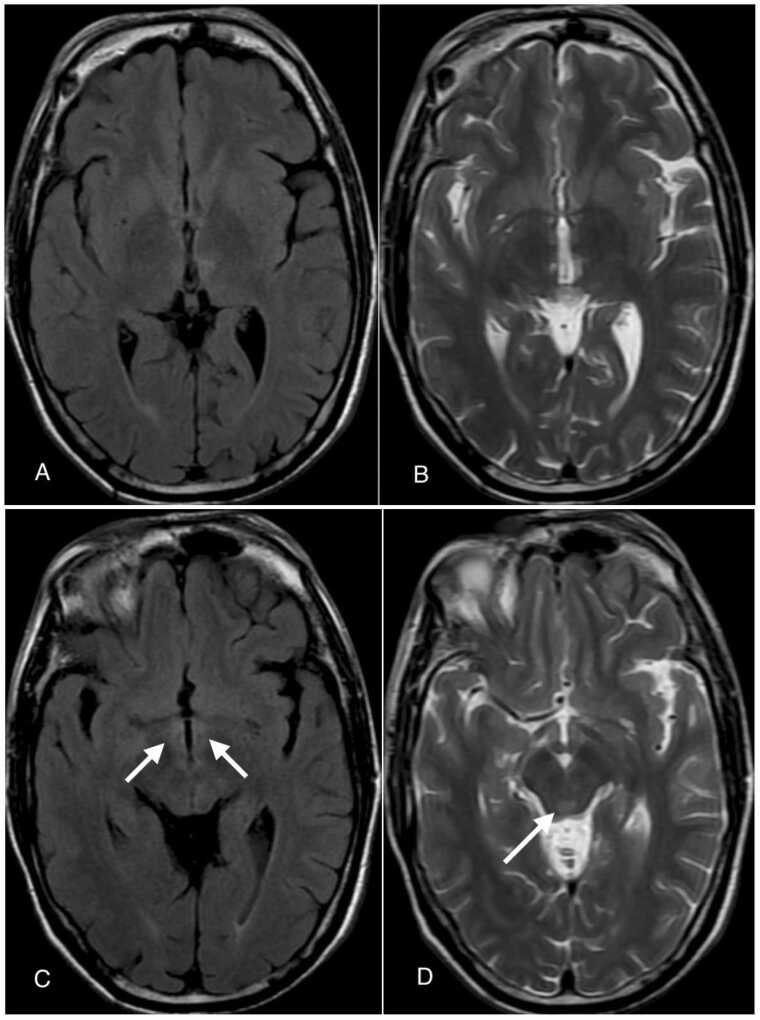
Axial T2-FLAIR (A and C) and T2-weighted (B and D) images showing decrease in the size of the previously seen altered signal intensity areas involving left thalamus and left cerebral peduncle with minimal T2 Flair hyperintensity seen in medial aspects of both thalami, mammillary bodies and in the periaqueductal region (white arrows in C and D).
